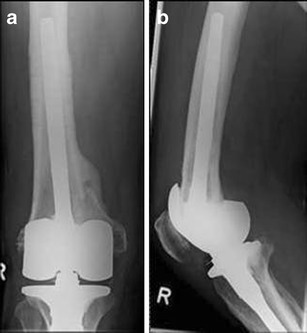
a, b Postoperative x-ray after implantation of a hinged revision TKA
